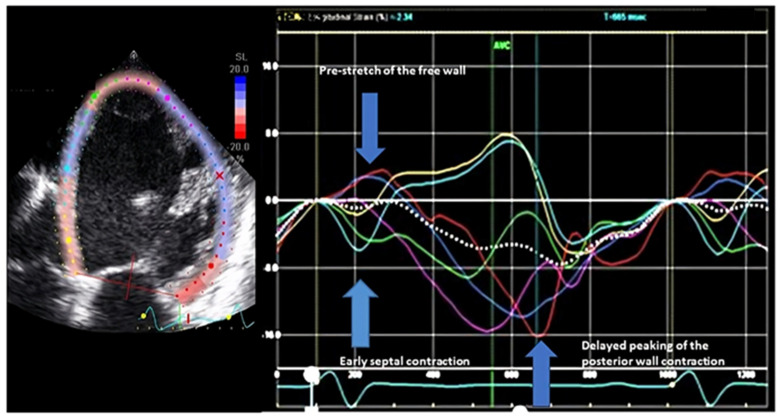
Typical contraction pattern using longitudinal strain. This is an example of a typical left bundle branch contraction pattern showing all three features. It is a simple qualitative technique performed using speckle tracking echocardiography by simply placing regions of interest on the LV in an apical four-chamber image.
